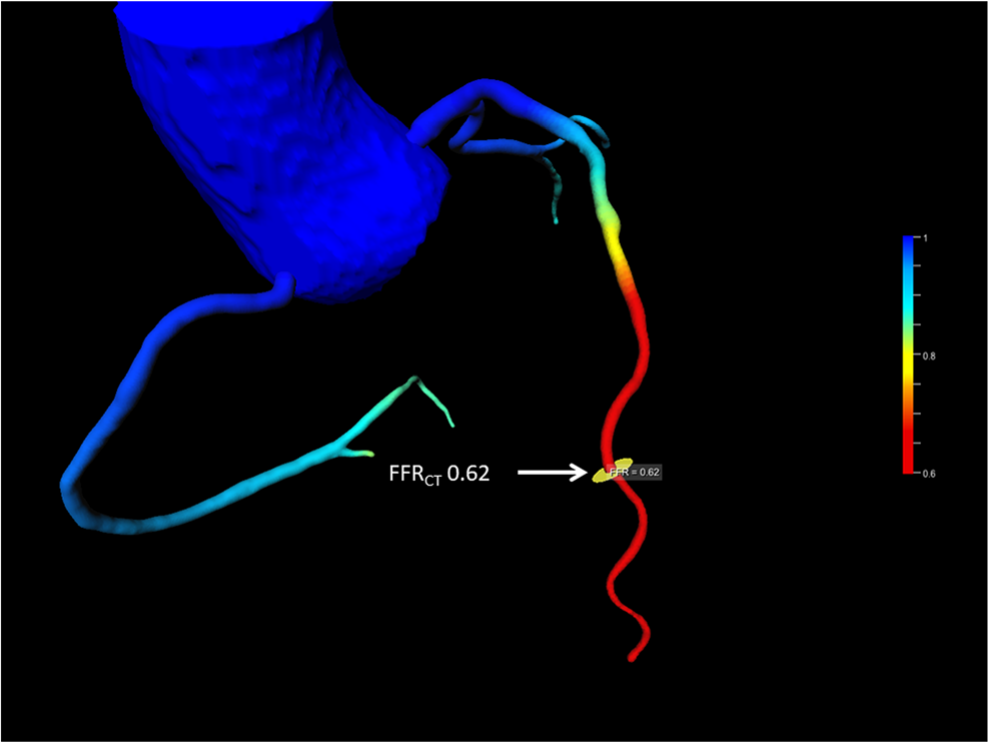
Example of FFR_CT_ evaluation without coronary stenosis. Despite the absence of coronary stenosis, the FFR_CT_ evaluation resulted in values of ≤ 0.8 in the distal section. The location of FFR_CT_ measurement is marked by an arrow. *Abbreviations: FFR*_*CT*_, CT fractional flow reserve
